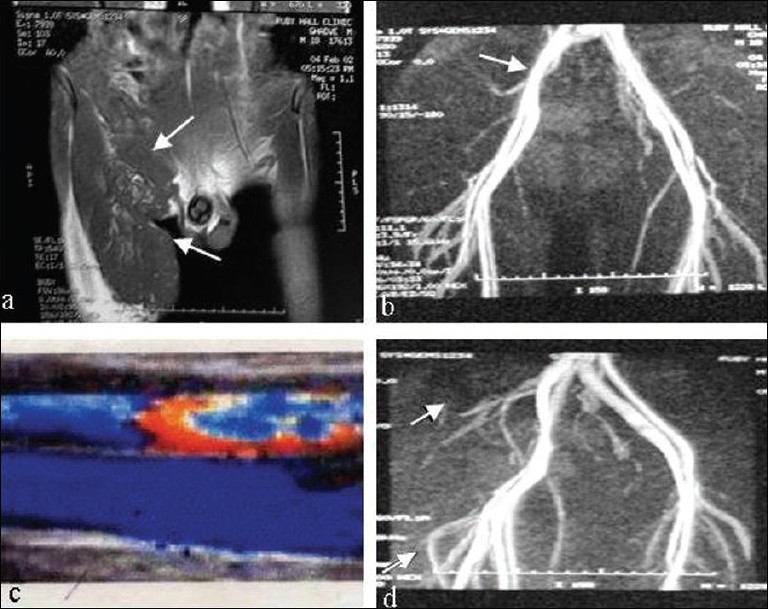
(a) MRI showing extensive mass in the subcutaneous tissue of right thigh with retroperitoneal extension. (b) MR angiogram showing narrowing of right iliac and femoral vessels due to extrinsic compression. (c) Colour Doppler showing normal caliber of vessels with optimum flow. (d) lymphangiography revealing dilatation of lymphatic channels in leg and inguinal region (Rt.).
